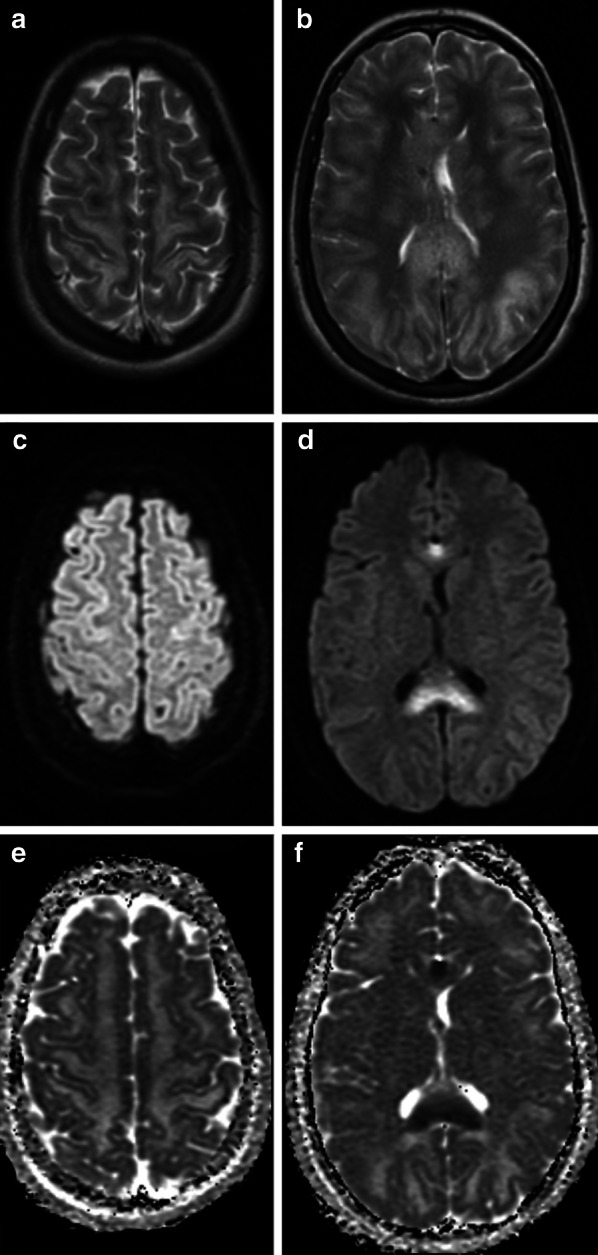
A case representing the various patterns of diffusion abnormalities seen in CFE. T2 (a, b), DWI (c, d), and ADC (e, f) MRI images at the level of the centrum semiovale (left column), and at the level of the basal ganglia (right column) of a 16-year-old polytraumatised female patient acquired on day 12 after hospital admission. T2 weighted images show hyperintense signal in the subcortical white mater, internal capsule and the corpus callosum (a, b). Diffusion-weighted MR Image with a b value of 1000 s/mm^2^ (c, d), and ADC (e, f) images show cytotoxic edema affecting the corpus callosum and facilitated diffusion over the subcortical white matter
